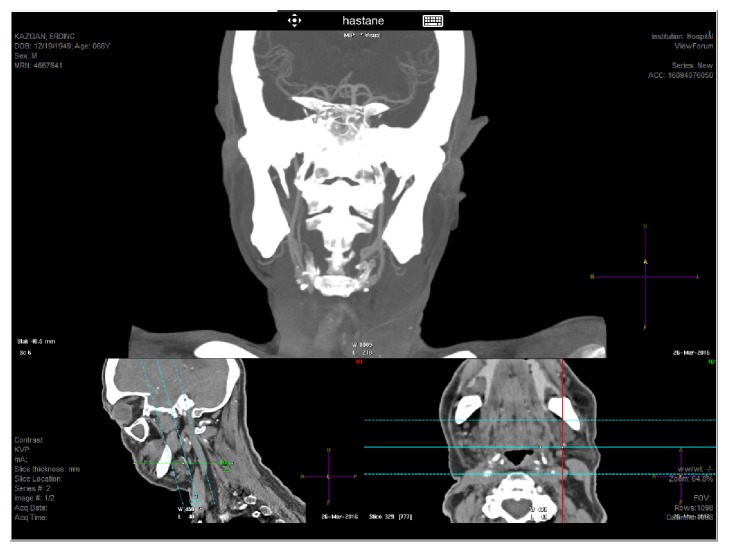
Coronal oblique reformatted computed tomography angiographic image through the neck shows occlusion of both common carotid arteries. Both internal and external carotid arteries are patent, however, filling via collateral flow. Intracranial carotid circulation and posterior circulation are patent.
